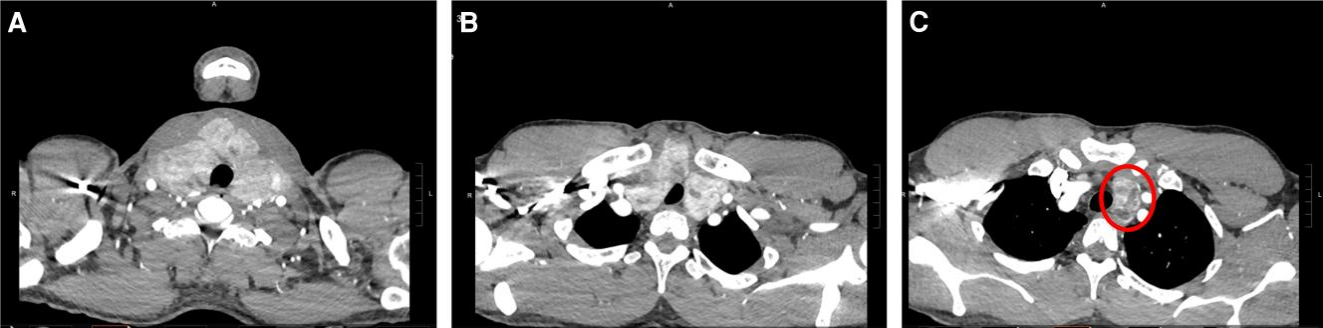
Computed tomography angiogram of the chest performed 4 months prior to radiofrequency ablation, when the patient presented with COVID-associated deep vein thrombosis. In the most superior axial image, the goiter is evident (A) and displacing the trachea posteriorly; (B) thyroid extends below the clavicles with tracheal narrowing; (C) Substernal extension from the left thyroid [circle].
